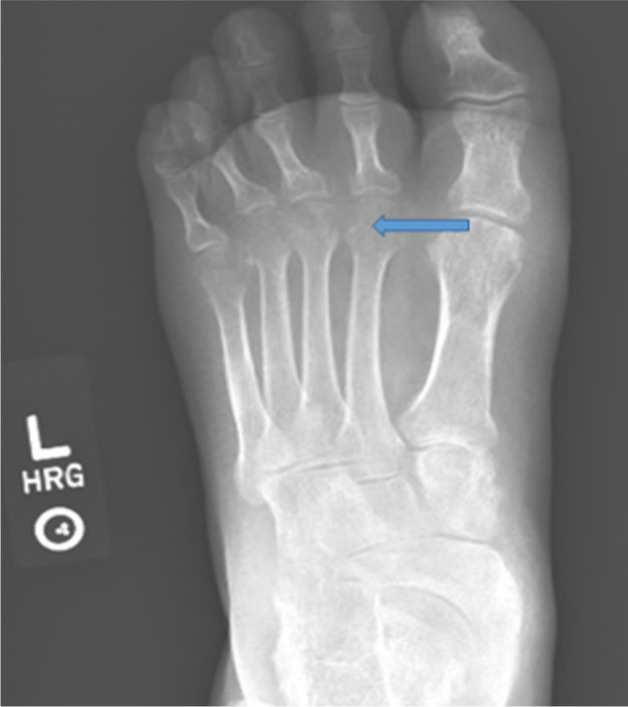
Foot X-ray showing metatarsal erosions.
